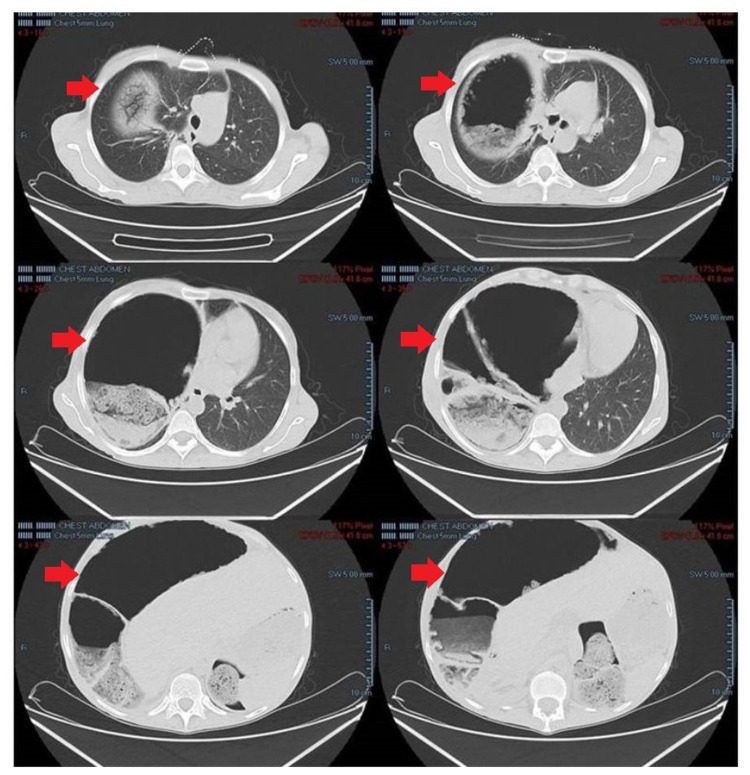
Abdominal CT shows the interposition of the right colon in the right hemithorax between the diaphragm and the liver without any signs of perforation (red arrows).
